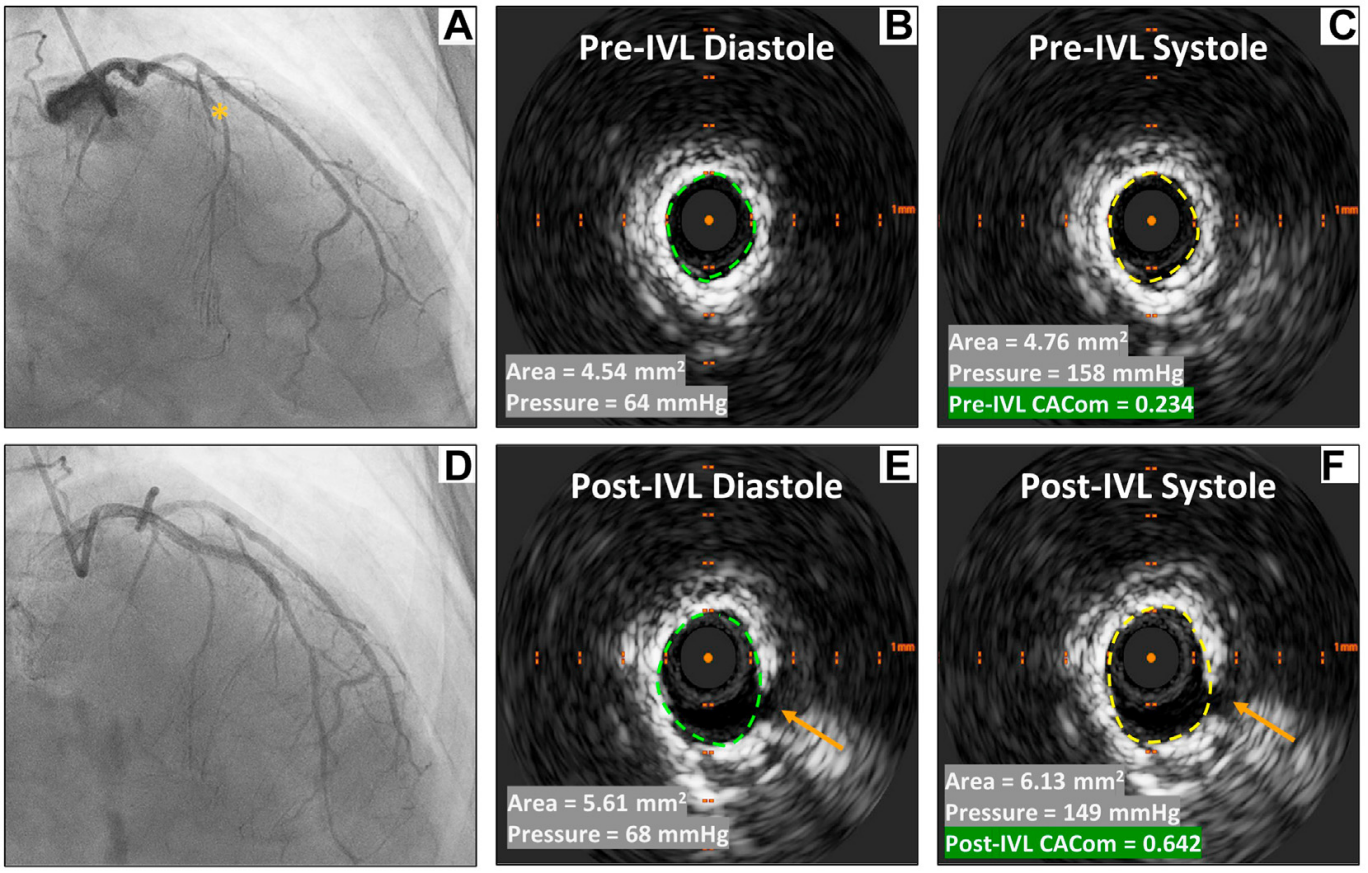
Coronary angiography and intravascular ultrasound images in a patient with anterior ST-elevation myocardial infarction. Occlusion in the mid–left anterior descending artery (asterisk) was assessed (A). Intravascular ultrasound revealed a long, 360° calcification in the culprit vessel. The luminal area was measured in diastole (B) and systole (C) before intravascular lithotripsy (IVL) pulse administration, while aortic pressure was recorded simultaneously. Pre-IVL coronary artery compliance was then calculated. A long, 4.0 × 34 mm drug-eluting stent was deployed (D). Before stenting, the luminal area and aortic pressure were measured again, both in diastole (E) and systole (F). Calcium fracture was assessed (arrow). Post-IVL coronary artery compliance was calculated.
